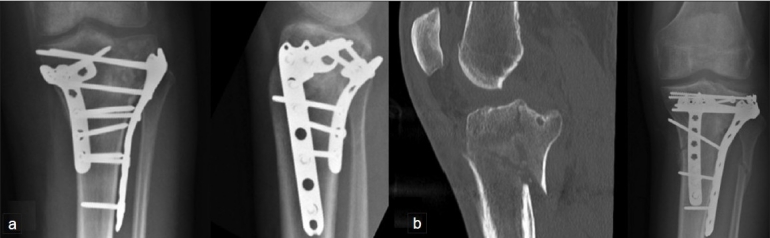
(a) Posteromedial plate and lateral plate fixation of a bicondylar fracture. (b) Sagittal CT scan showing the apex of the posteromedial fragment which was stabilized with a posterior plate followed by lateral plate stabilization
